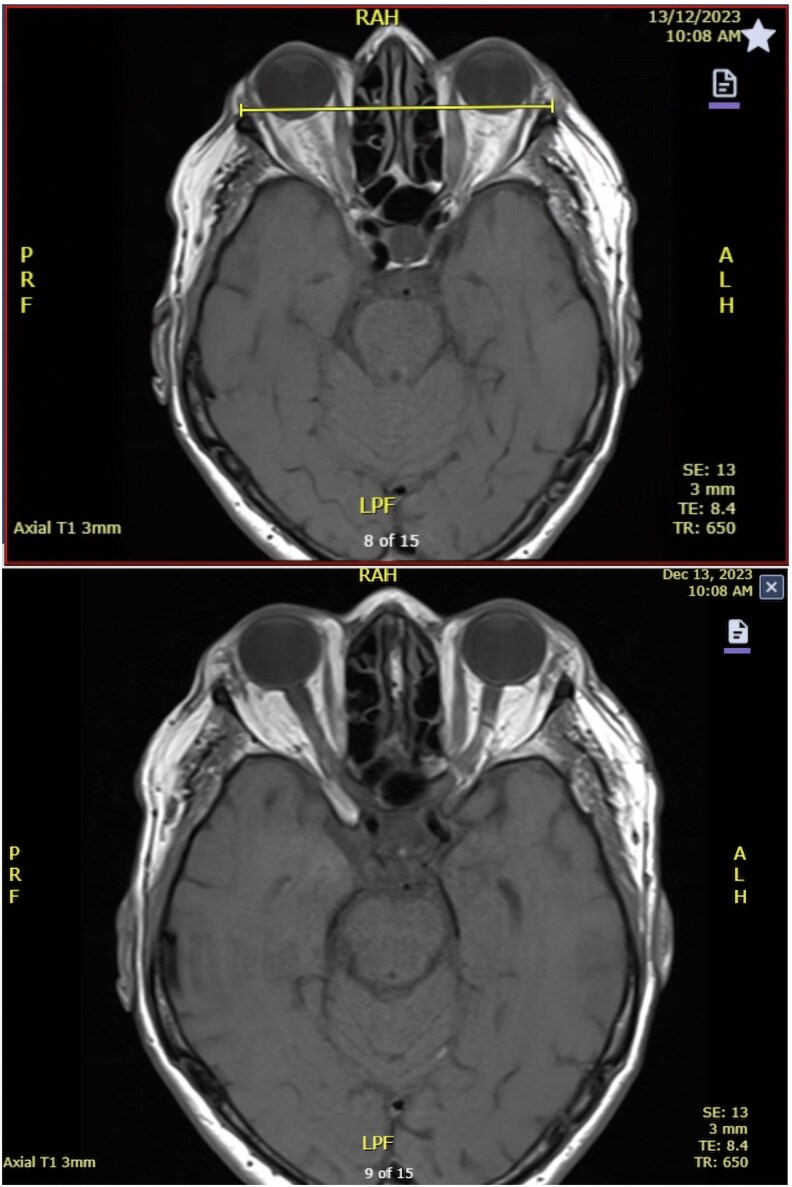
Axial magnetic resonance imaging showing symmetric bilateral exophthalmos and bilateral enlargement of the extraocular muscles, most prominently involving the inferior and medial rectus muscles.
